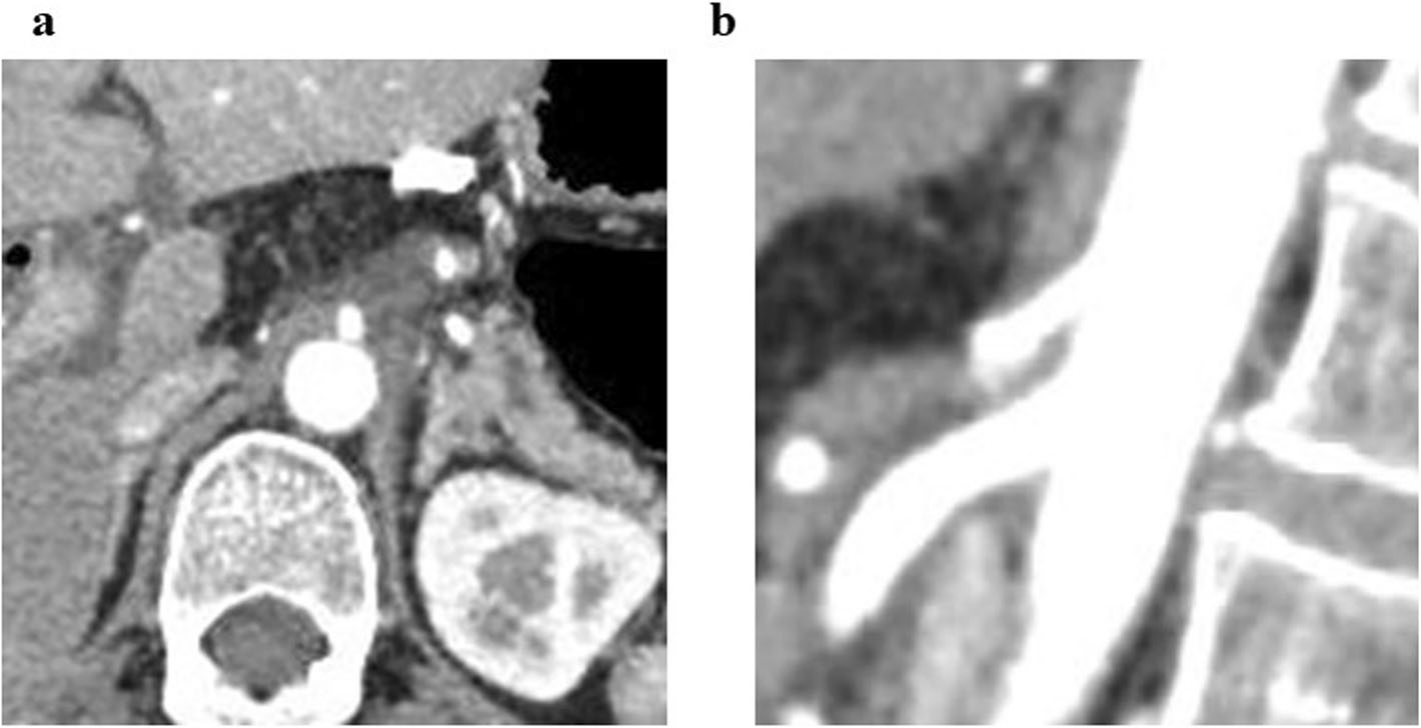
CT at postoperative day7 (case 3). Disappearance of celiac artery stenosis
